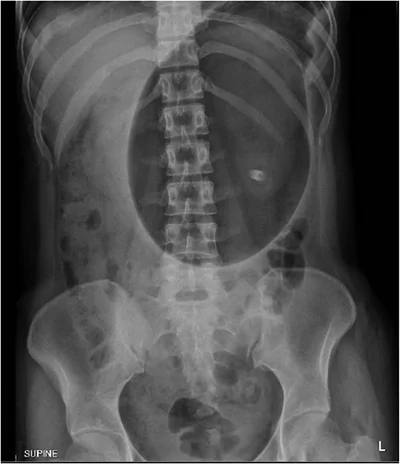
Abdominal radiograph (supine) demonstrating a grossly distended intragastric balloon with an air‐fluid level, without evidence of intestinal obstruction or free perforation.
